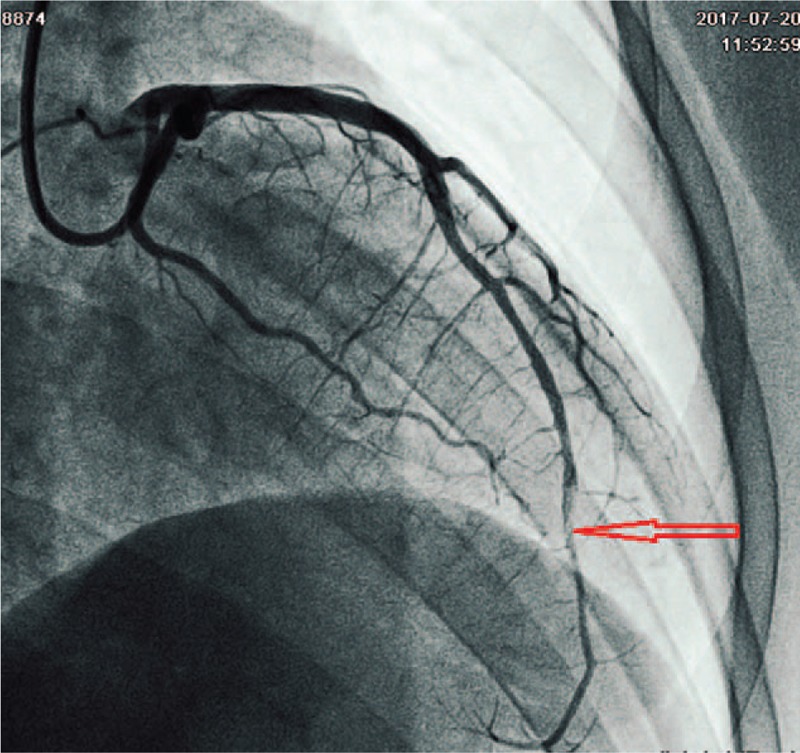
Echocardiographic examination revealed a moderate echogenic mass at the apex.
